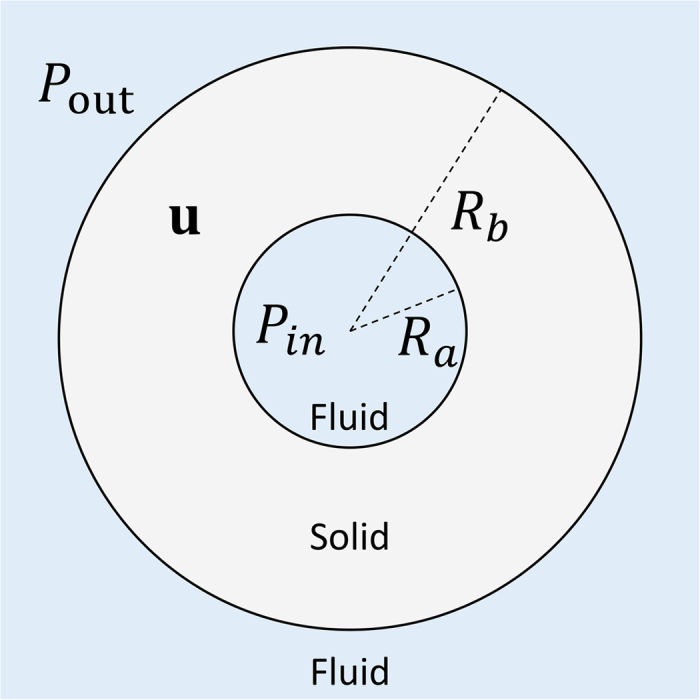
Structure of our 2D shell cavity. It consists of three sub-regions: inner fluid, a solid shell, and outer fluid. Ultrasound fields are described in terms of pressure *P*_in_ and *P*_out_ inside the inner and outer fluid and displacement u inside the solid.
